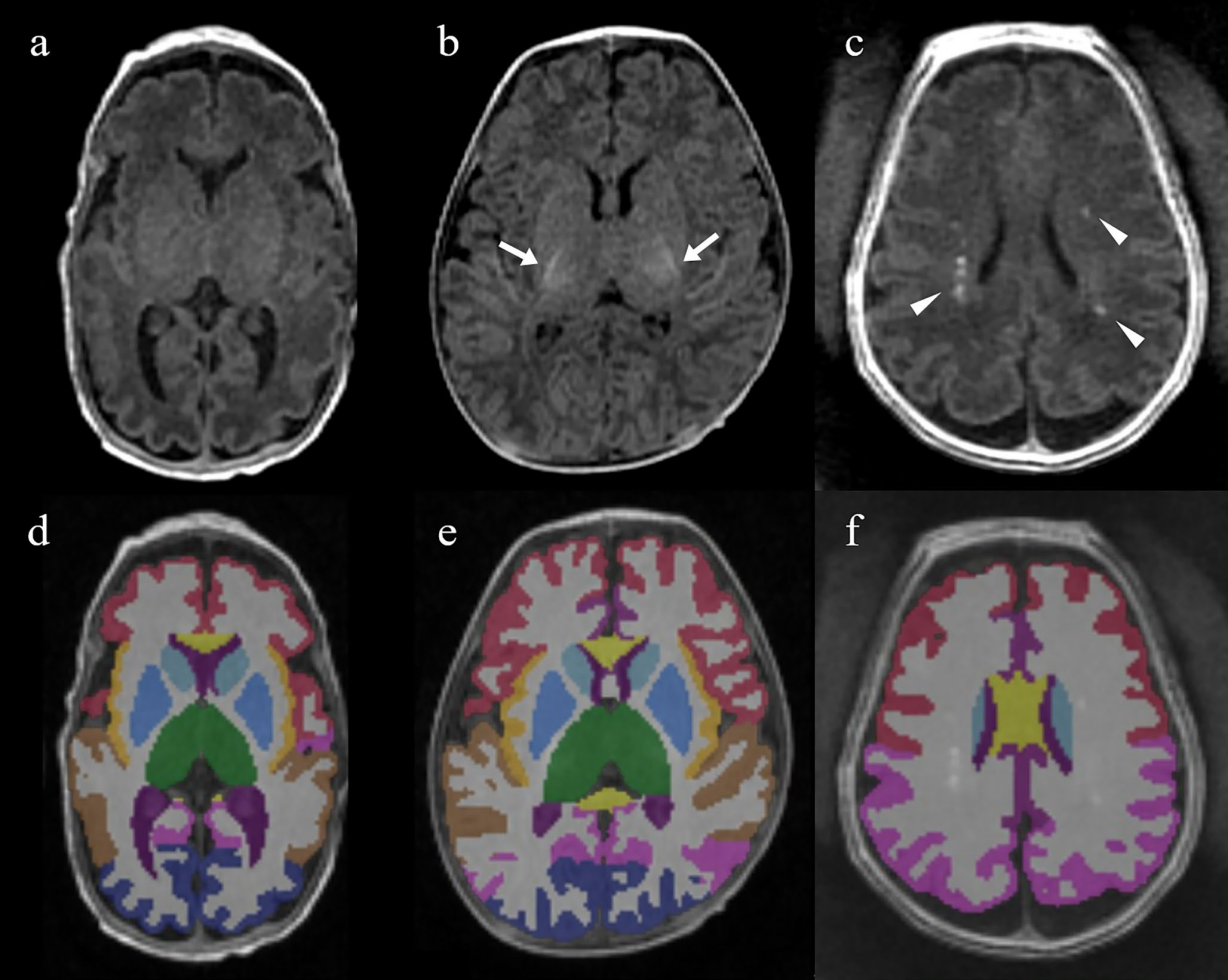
Representative T1-weighted brain magnetic resonance imaging (a–c) and corresponding segmentation images (d–f) across different myelination stages and in punctate white matter lesion (PWML). (a, d) Male infant born at 26 weeks’ gestation and imaged at postmenstrual age (PMA) 35 weeks. (b, e) Male infant born at 35 weeks’ gestation and imaged at PMA 45 weeks, showing myelination in the posterior limb of the internal capsule (arrows). (c, f) Female infant born at 29 weeks’ gestation and imaged at PMA 36 weeks, showing bilateral PWML in the periventricular white matter (arrowheads). Myelinated white matter and PWML were correctly segmented as white matter. In the segmentation image, red, pink, brown, blue, purple, yellow, violet, sky blue, green and gray correspond to the frontal lobe, parietal lobe, temporal lobe, occipital lobe, cingulate cortex, insula, lateral ventricle, caudate, thalamus, and white matter, respectively. PWML, punctate white matter lesion; PMA, postmenstrual age
